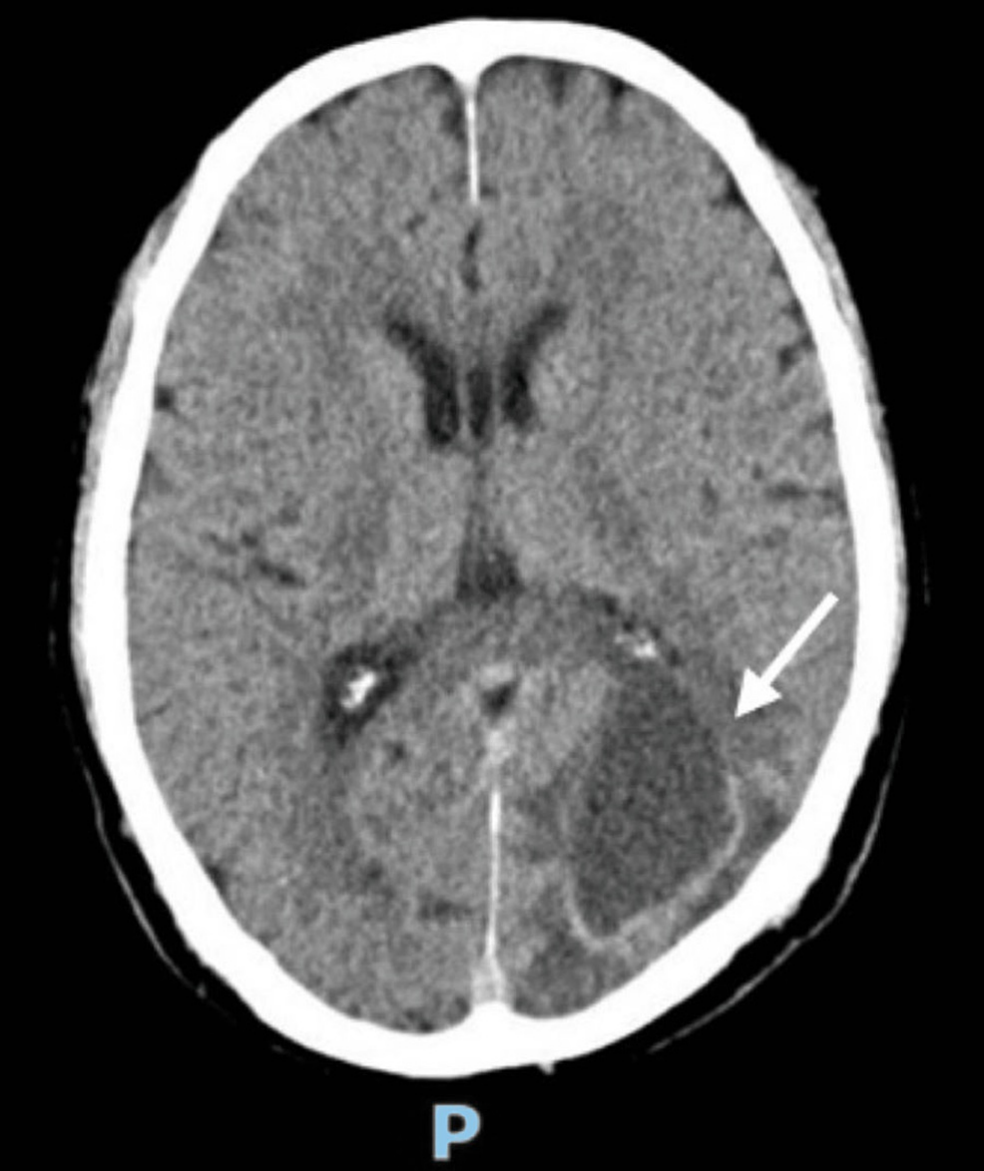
Axial cut of the head CT with visualization of left parieto-occipital lesion before surgery CT: computed tomography
